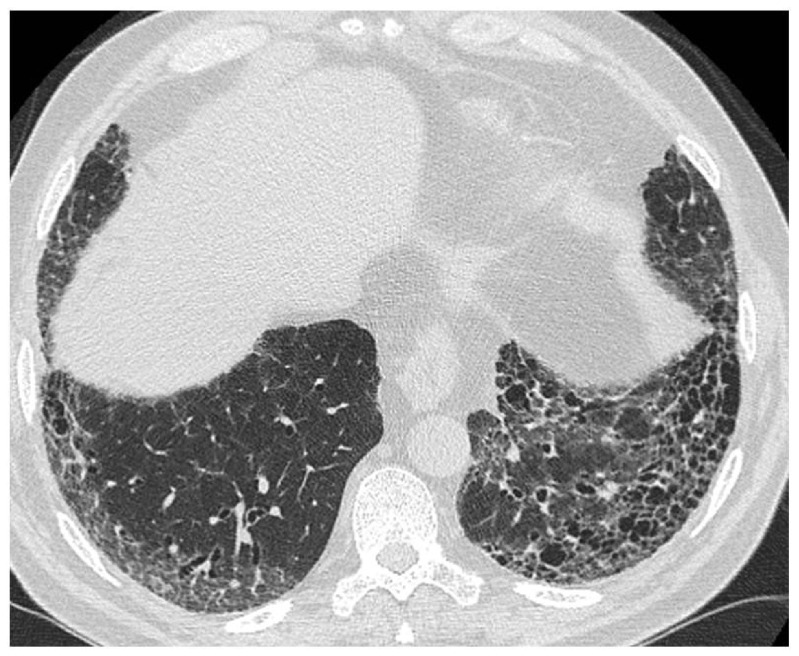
Typical high-resolution computed tomography (HRCT) pattern of usual interstitial pneumonia (UIP). The image shows subpleural and basal predominance of reticular opacities associated with traction bronchiectasis and honeycomb change (clustered cystic airspaces with well-defined thick walls and diameter of 0.3–1.0 cm).
